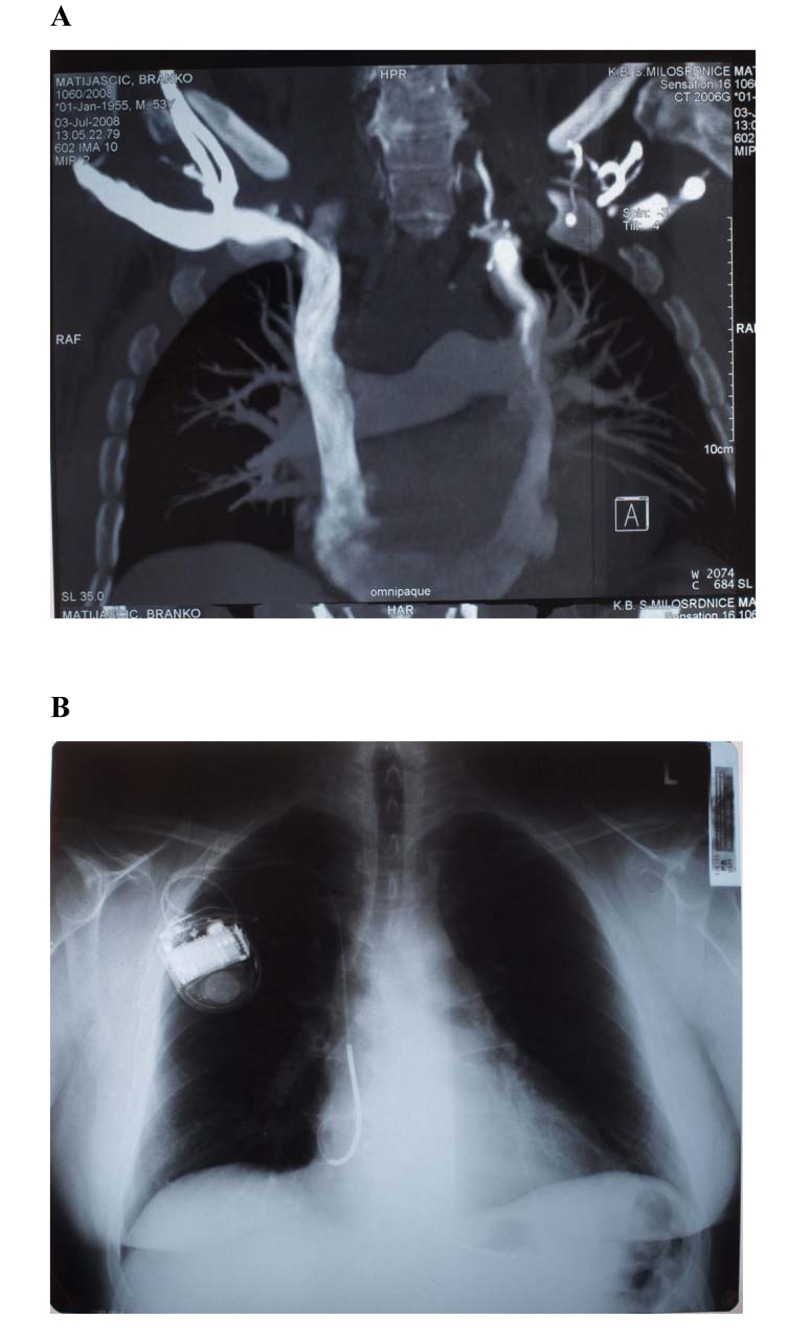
A) Multislice CT-angiography showing a persistent left superior vena cava with a presentation of right subclavian vein. B) A quadripolar dual coil active defibrillation lead was inserted in the right ventricle via right subclavian vein and connected with an ICD impalanted at right pectoral side (patient 3).
